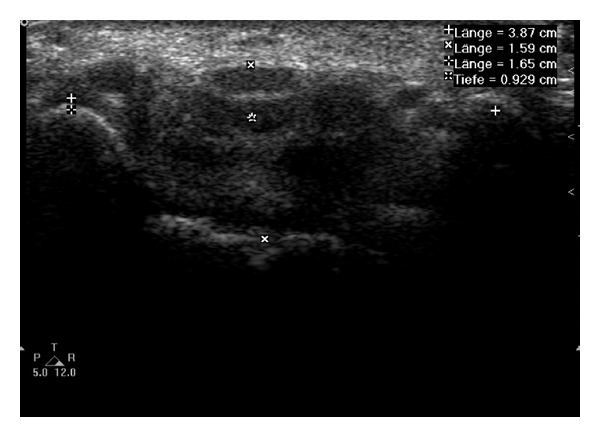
Internal wrist measurement: the radio-ulnar (width) distance between the pisiform (+) and scaphoid tubercle (+) and the dorsopalmar (depth) distance between the flexor retinaculum above the median nerve (*x*) and the lunate bone (*x*) were measured sonographically in the carpal tunnel.
